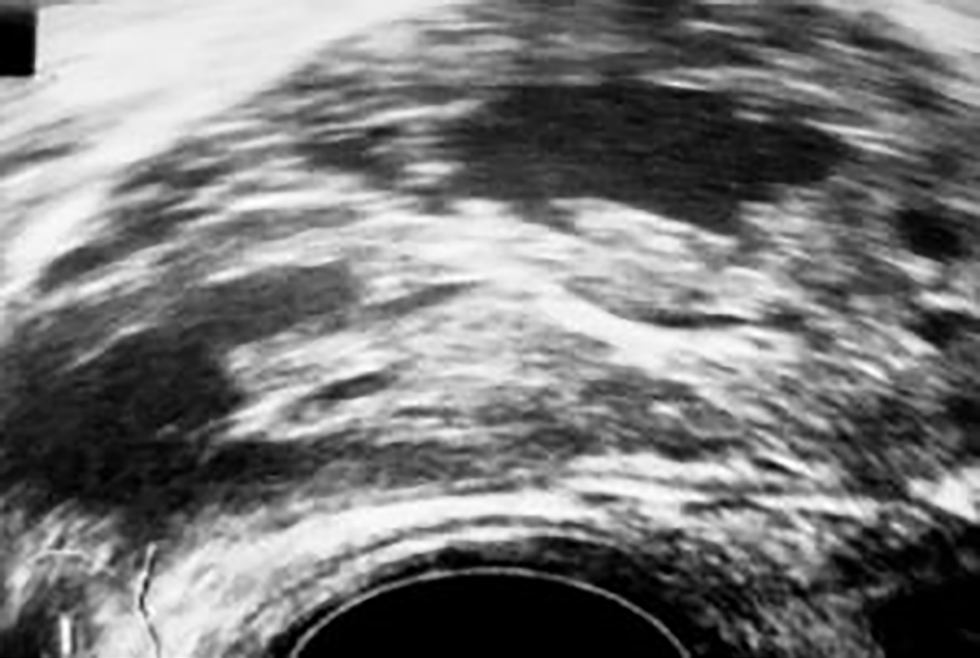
Ultrasonography of the ovarian tumor.
